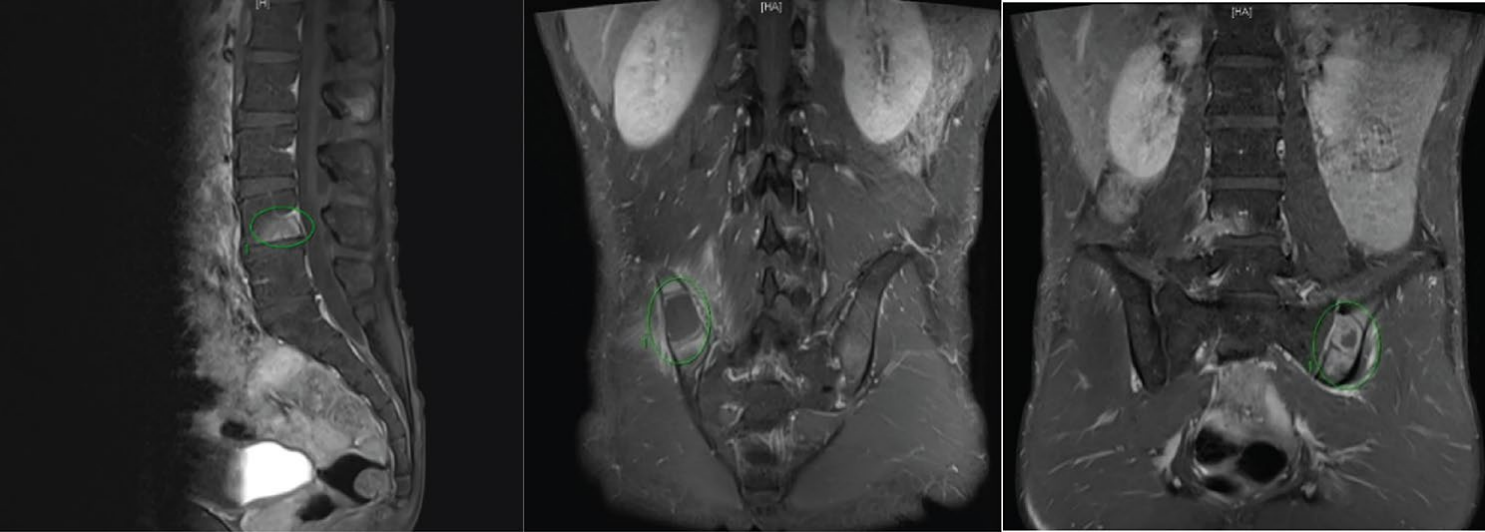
Magnetic resonance imaging (MRI) of the sacrum revealing diffuse decreased T1 signals affecting the thoracolumbar spine and bilateral iliac bones, along with multiple T2 hyperintensity lesions showing enhancement in the bilateral iliac bones and lower L4 vertebral body
